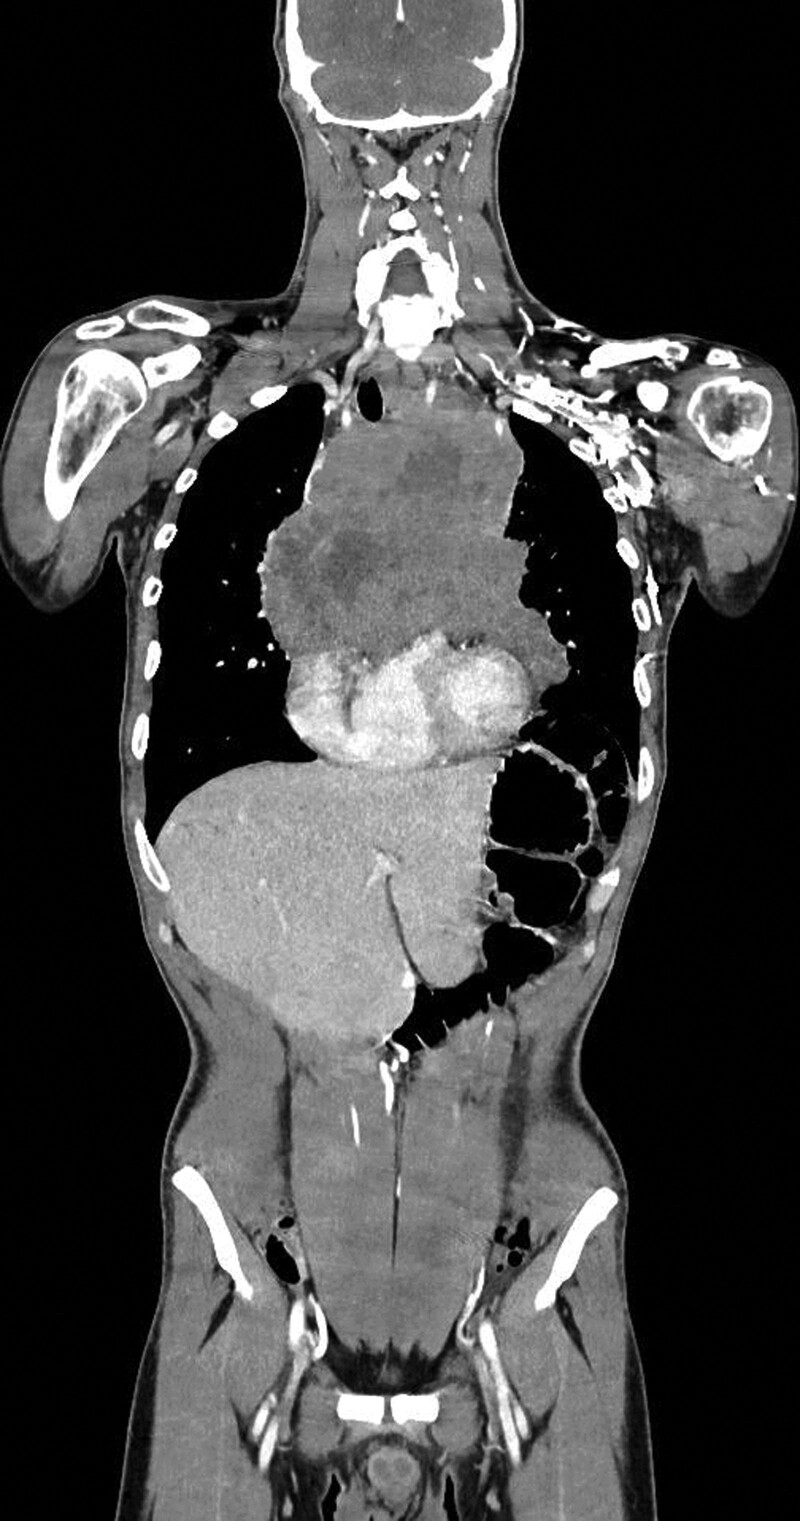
CT scan (coronal and axial axis) showing a solid antero-superior mediastinal vascularized mass (16 × 13 cm) encasing the great thoracic vessels.
